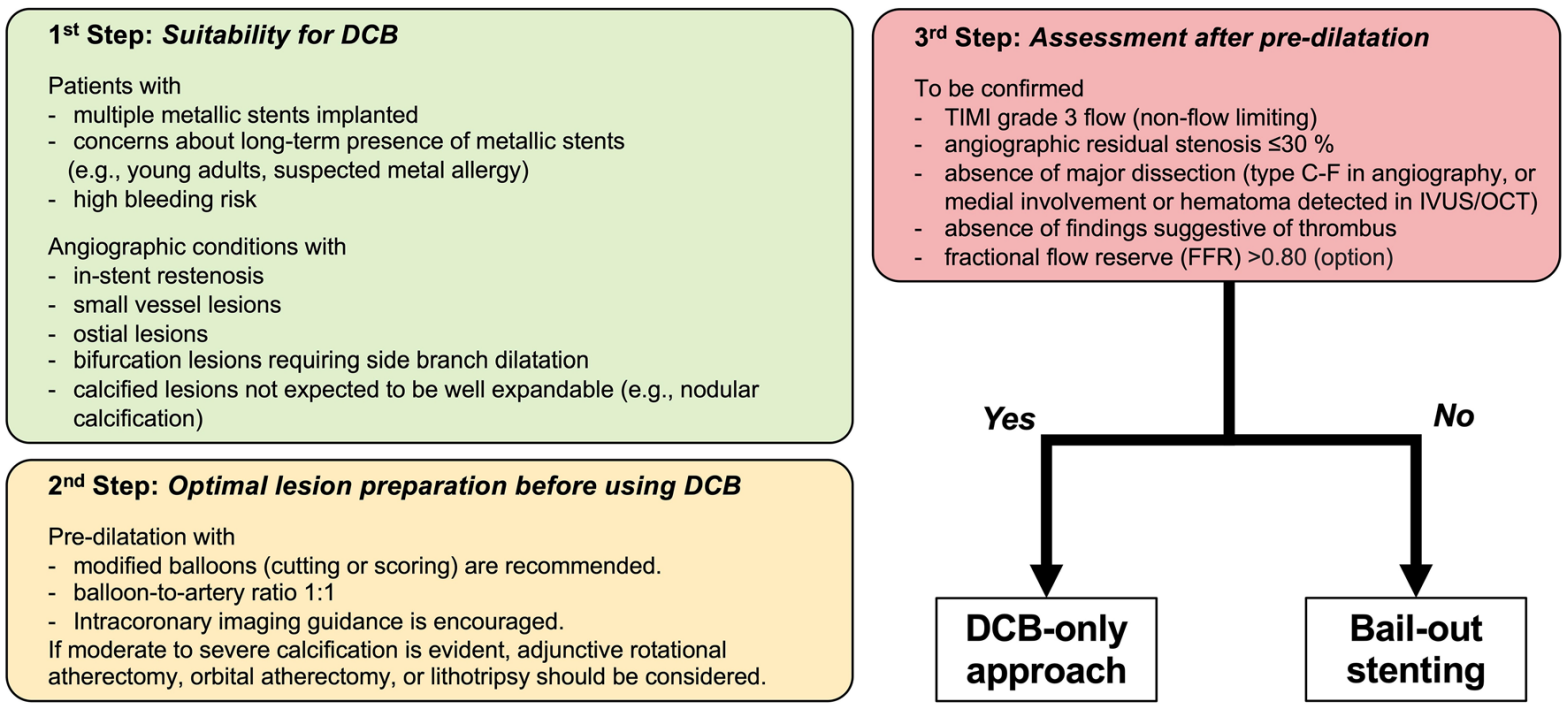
A proposed step-by-step approach for the DCB-only strategy. For possible indication of large vessel (≥ 3.0 mm) lesions, refer to an official statement of the CVIT issued on 1st Dec, 2022. (https://www.cvit.jp/_assets/documents/news/2023/0104.pdf). DCB, drug-coated balloon; IVUS, intravascular ultrasound; OCT, optical coherence tomography; DES, drug-eluting stent
